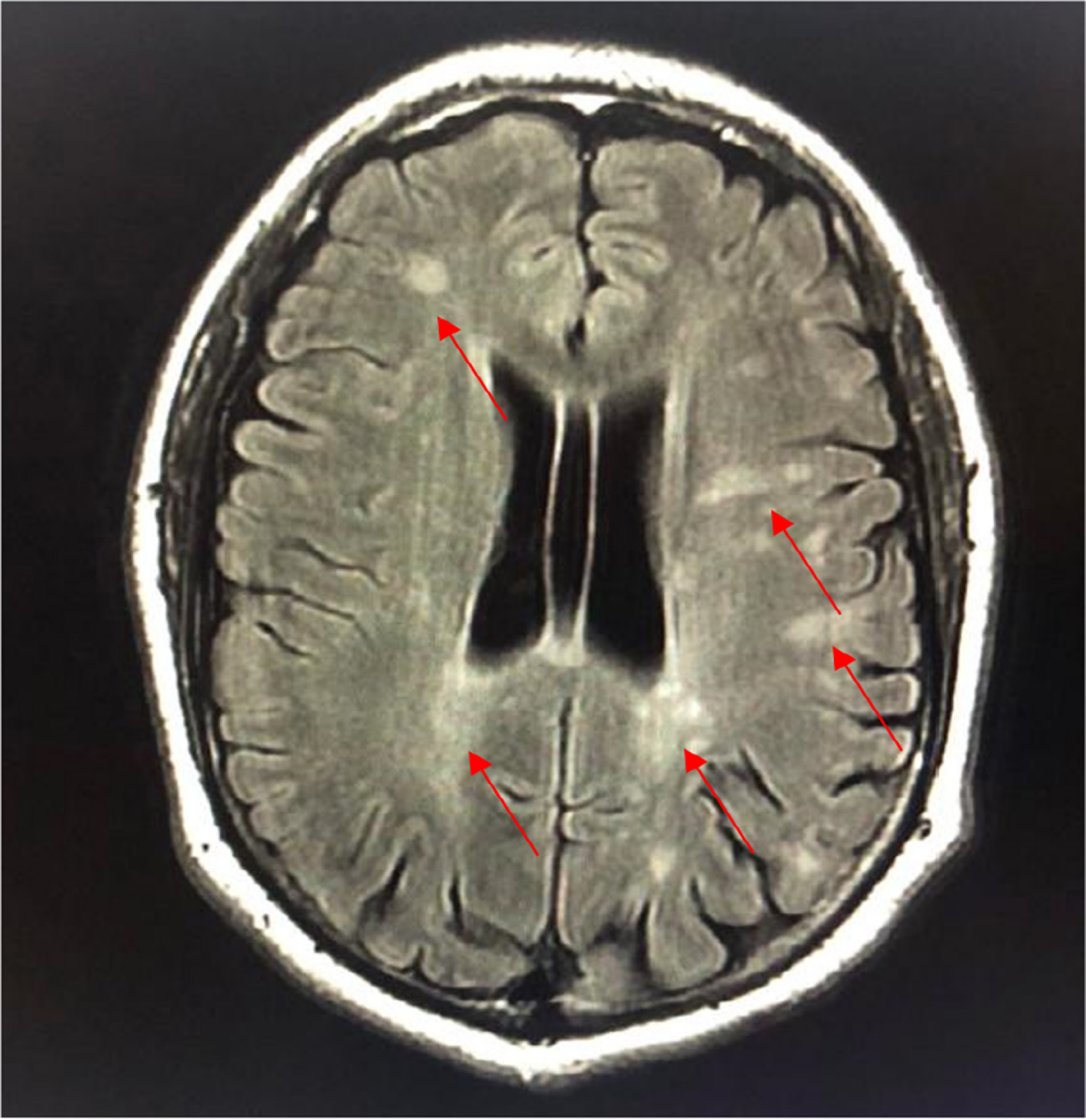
T2 FLAIR MRI of the brain in December year X+1 showing bilateral periventricular, right frontal oivoid subcortical and a lesion, which is perpendicular to the corpus callosum axis and parallel to the U fibers.
